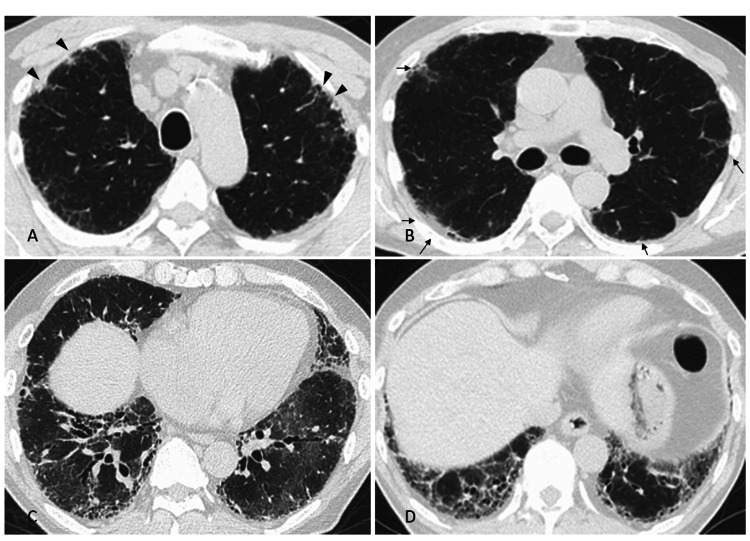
Chest computed tomography images (A, B) In the upper-lung zone, bilateral reticulation (arrows) and irregular thickening along the pleura (arrowheads) were shown. (C, D) Reticular shadows and ground-glass opacities were present predominantly at the base of both the lungs.
